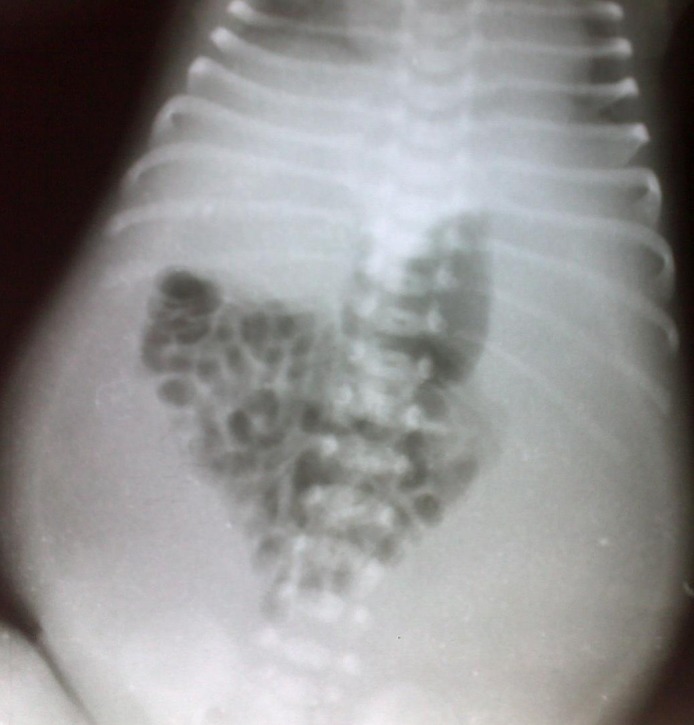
Figure 1: Abdominal radiograph.
